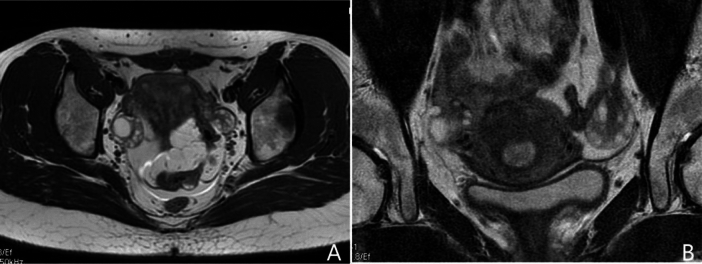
Current challenges in follicle counting using traditional fast spin-echo T2-weighted MR imaging. Panel (A) shows the full field of view image, which offers relatively high temporal resolution. However, its low spatial resolution makes follicle counting challenging. In contrast, panel (B) presents a small field of view image with higher spatial resolution, but it is prone to noise and motion artifacts due to its lower temporal resolution, which limits accurate follicle counting.
